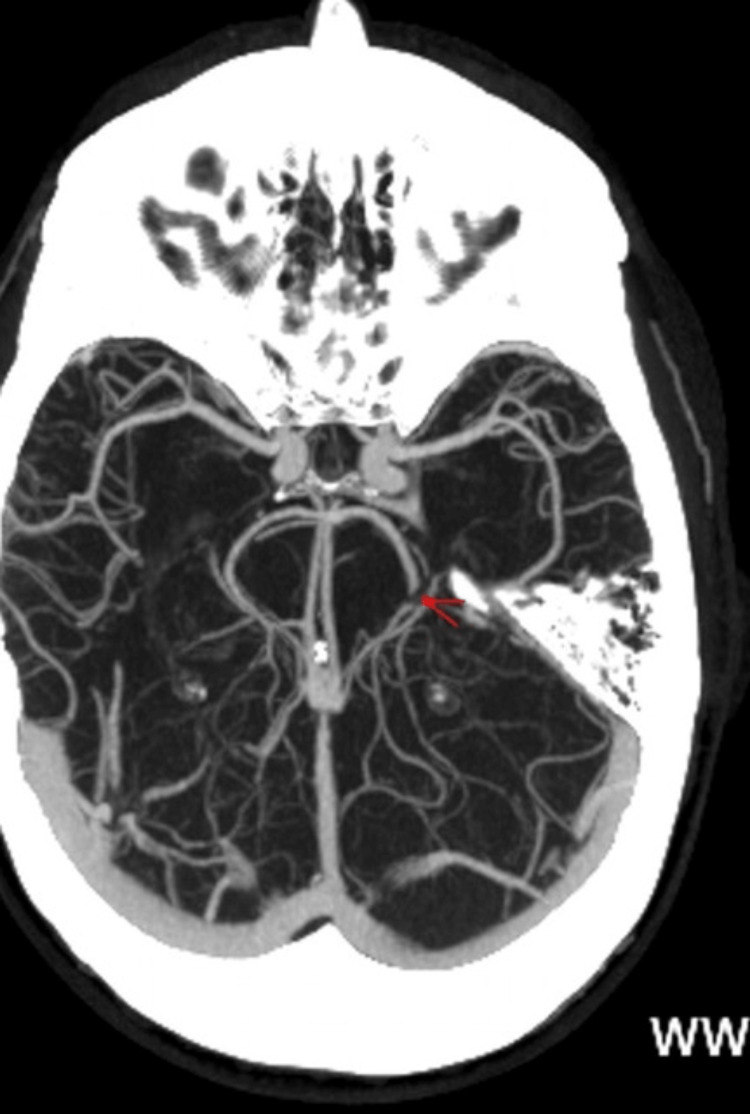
CT angiogram demonstrating a filling defect with abrupt cut-off of flow in the left PCA P3 segment with reconstitution of flow distally PCA: posterior cerebral artery
